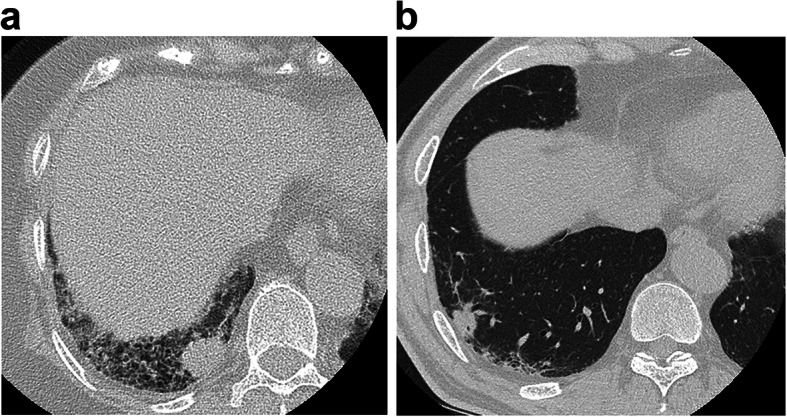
Lung cancer in midst of fibrotic shadow and/or interface of fibrotic shadow and normal lung. These are examples of computed tomography findings for lung cancers in the fibrotic shadow
